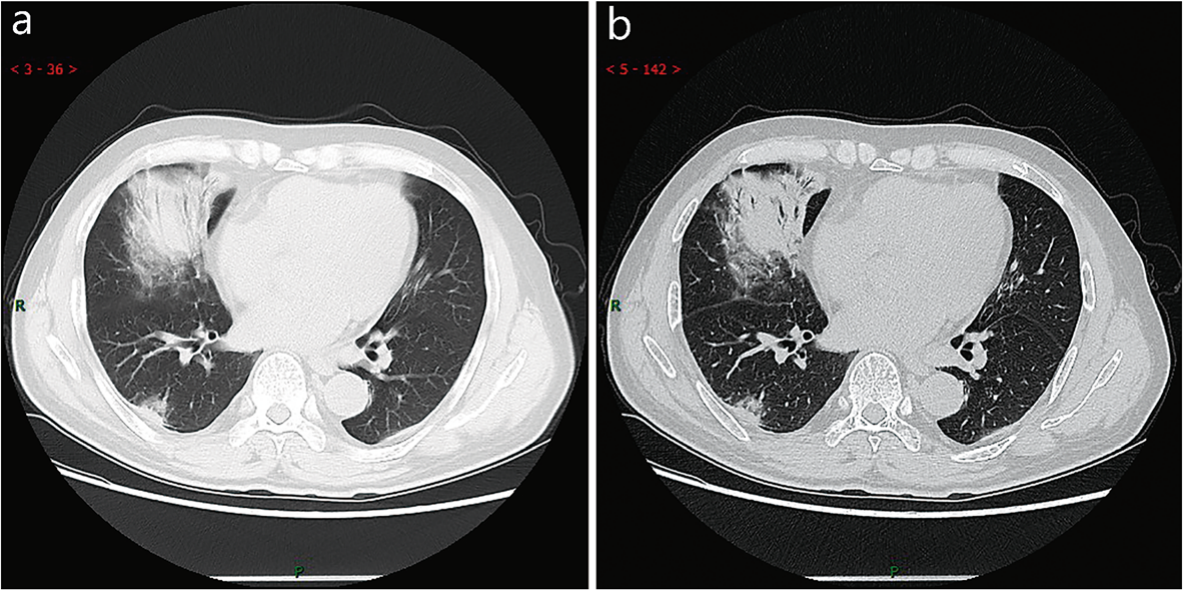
CT imaging of consolidation stage. A 65 years old male with fever (maximum temperature of 39 ℃). Laboratory test: hypoproteinemia (decreased total protein (62.20 g/L), decreased albumin (35.70 g/L)), abnormal liver function (increased alanine aminotransferase (79 U/L), increased aspartate aminotransferase (72 U/L)), increased procalcitonin (0.10 ng/ml), increased C-reaction protein (53 mg/L), decreased white blood cells (3.72 × 10^9^/L), decreased lymphocytes (0.9 × 10^9^/ L), mildanemia (decreased red blood cells (4.10 × 10^12^/L), decreased hemoglobin (131.10 g/L), decreased hematocrit (39.0%). Imaging examination: a (thin layer CT) and b (high-resolution CT) showedmultiple patchyand large consolidation in right middle lobe, posterior and basal segment of right lower lobe and outer and basal segment of left lower lobe, with air-bronchogram inside
